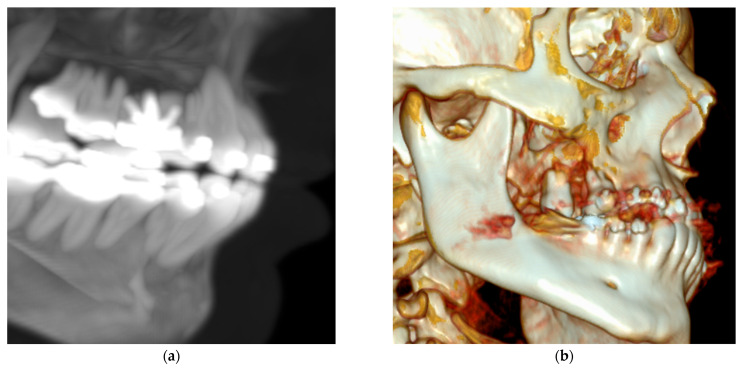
Contrast-enhanced computed tomography (a) Three-dimensional reconstruction with MIP mask showing the endodontic treatment performed on the upper first molar. (b) Three-dimensional reconstruction showing bone loss/erosion in the right maxilla, primarily affecting the molars.
